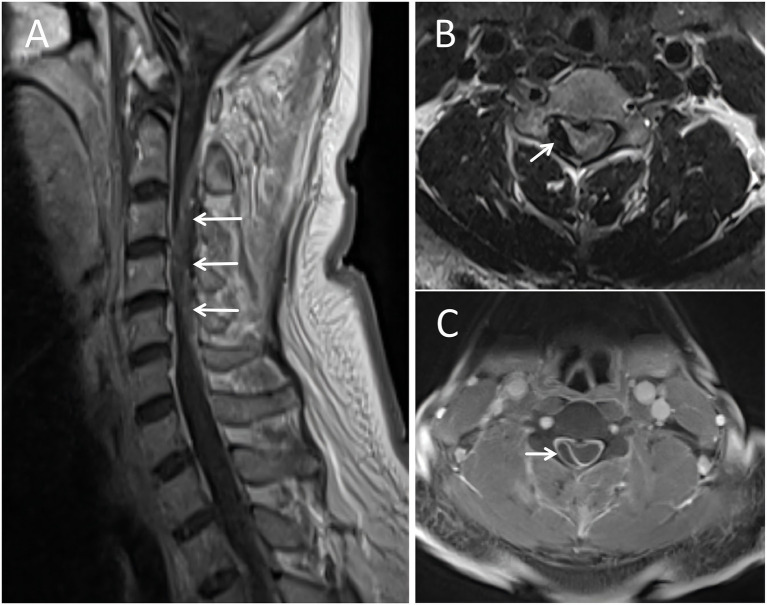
Cervical spine MRI. (A) Sagittal T1-weighted image demonstrates a slightly hyperintense signal within the mass in the dorsal epidural space from C2 to C6 (white arrows). (B) Axial T2-weighted image reveals a hypointense mass causing spinal cord compression (white arrow). (C) Axial contrast-enhanced T1-weighted image shows no enhancement of the mass (white arrow), confirming the absence of vascular malformation or neoplasm.
